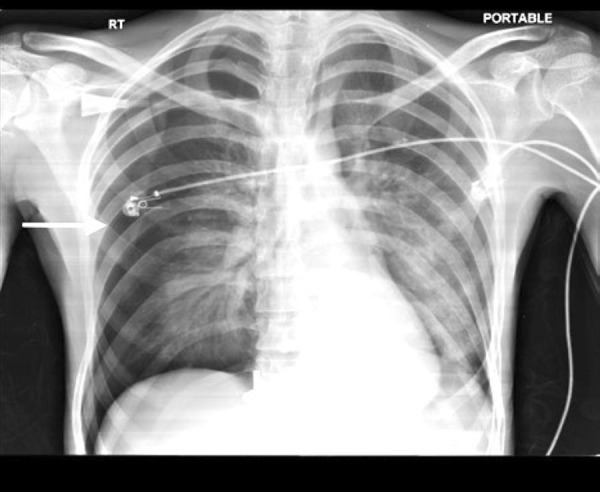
Chest x-ray showing right-sided pneumothorax.
